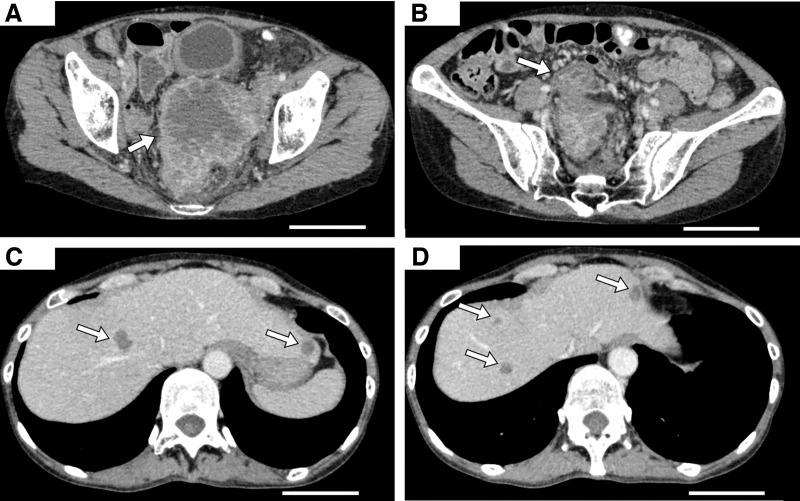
CT findings on day 7 after pembrolizumab (scale bar, 10 cm). (A) Rapid enlargement of the primary tumor (103 × 81 mm) with abscess formation was observed; the arrow indicates the enlarged primary tumor. (B) Newly developed lateral lymphadenopathy was noted; the arrow indicates the lateral lymph node. (C, D) Multiple new liver metastases were identified; the arrows indicate the newly developed liver metastases.
